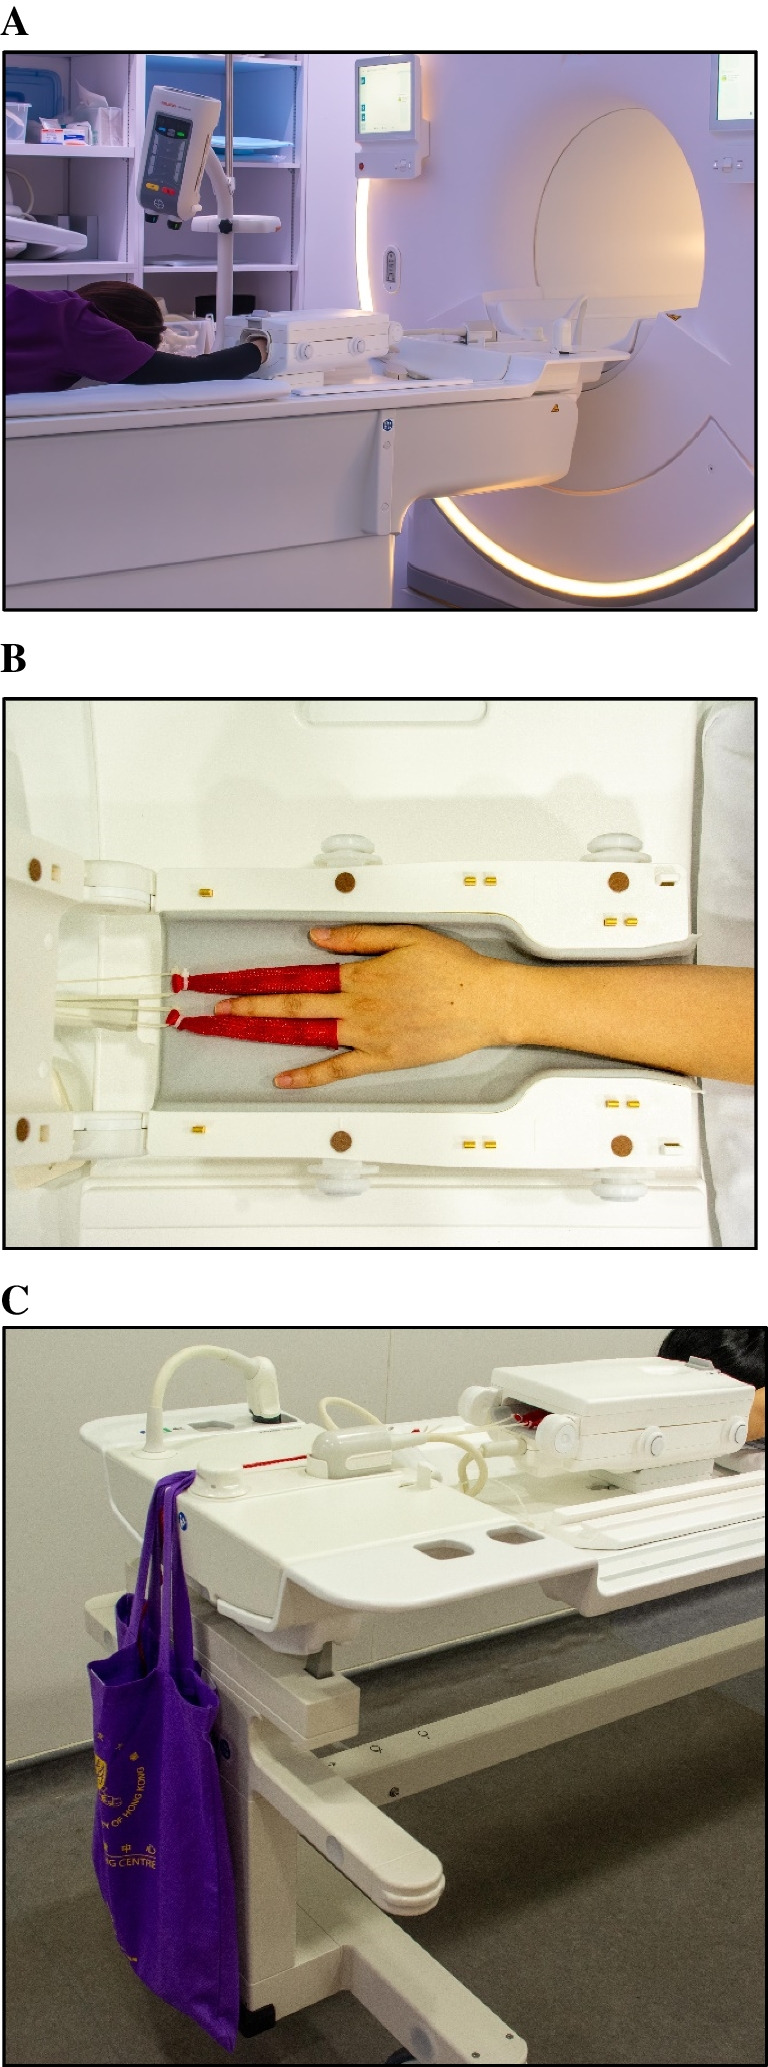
Patient positioning for wrist MRI with traction. (A) Patient lies in a ‘Superman’ position, with (B) the index and ring fingers entrapped in Chinese finger traps, (C) connected to suspended weights via a non-elastic cord
